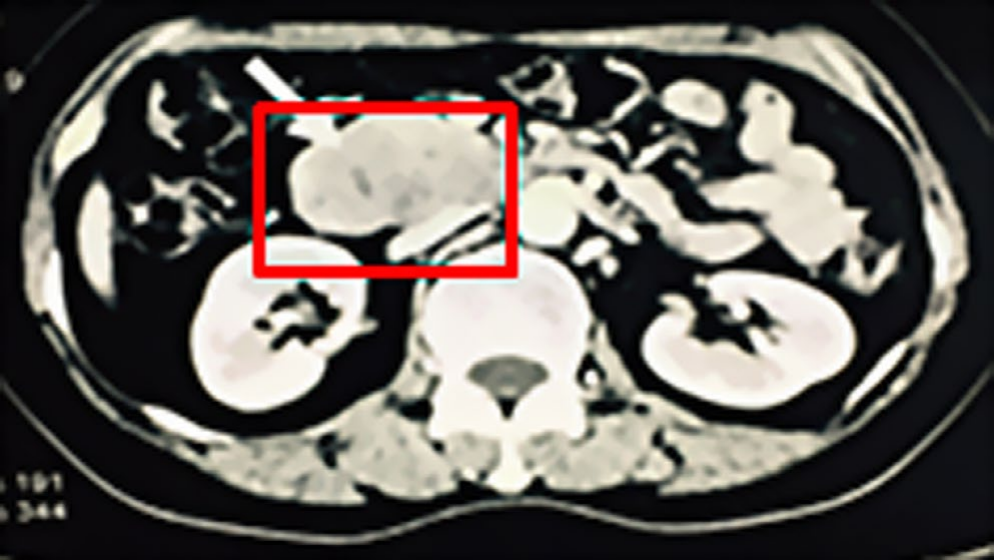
CT scan showed a mass in ampullary and head of pancreas
